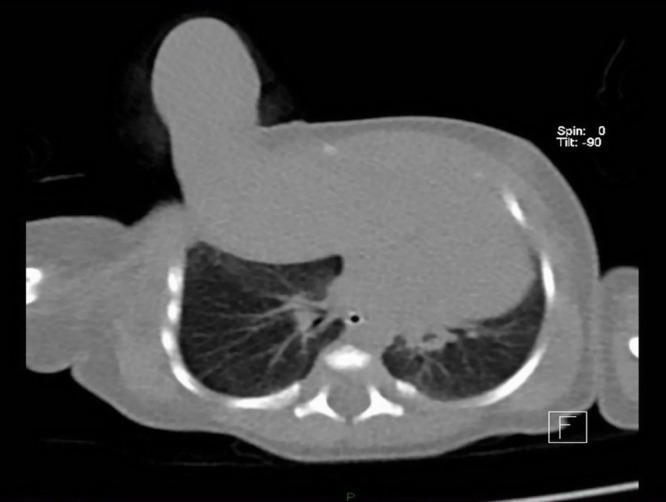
Figure 2: A) CT scan of liver showing liver protrusion through the thoracic wall defect.
